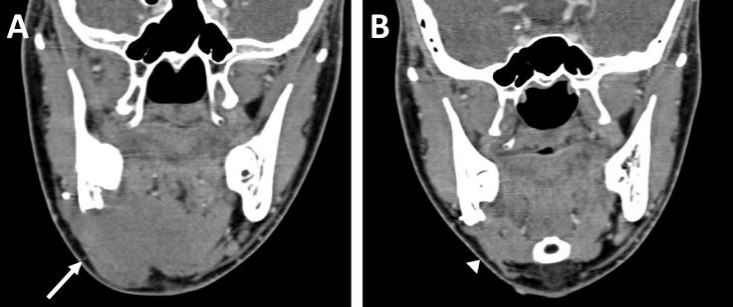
Hard Swelling in the Right Submandibular Region of an 18-year-old Patient. A: Pre-chemotherapy CT in coronal reconstruction shows homogeneous mildly enhancing lesion in the right submandibular region and floor of mouth (arrow, A). B: Post-chemotherapy CT shows significant decrease in lesion size (arrowhead, B).
